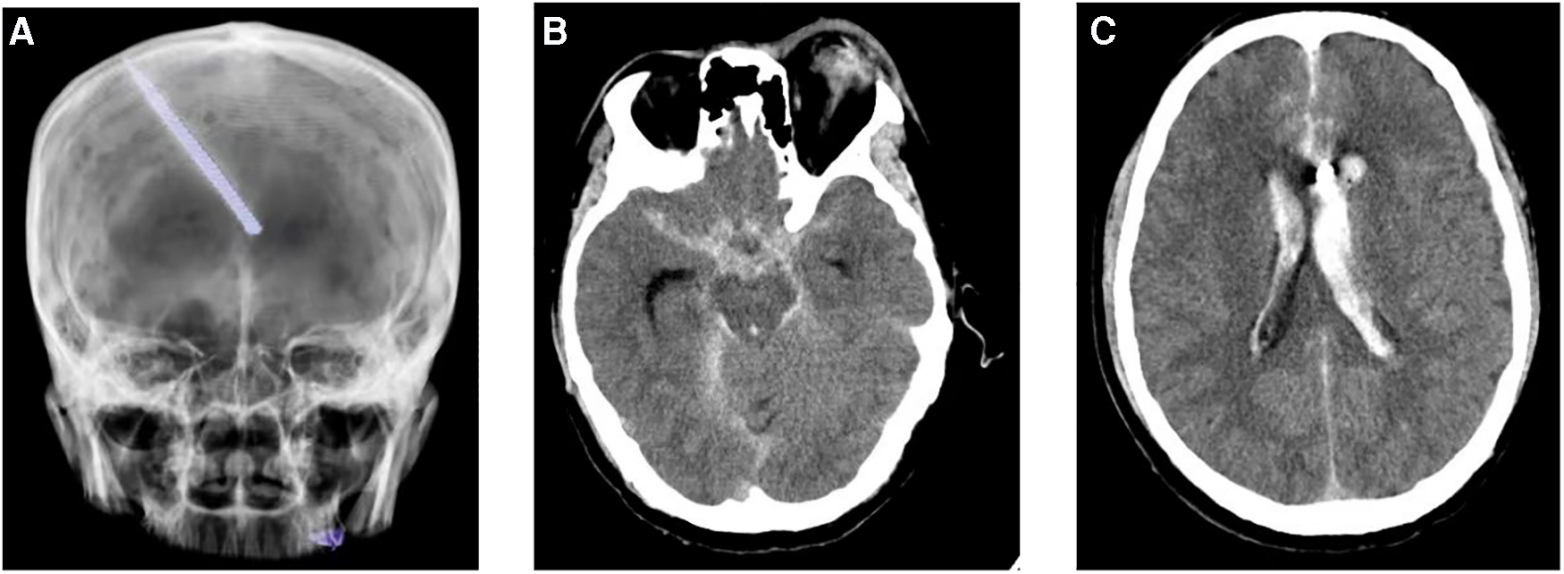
Computed tomography reveals a foreign body (metal strip) spanning the genu of the corpus callosum to the right parietal lobe (A); rupture of the left eyeball, subcutaneous hematoma of the eyelid, fracture of the top wall of the left orbit, and a small accumulation of gas in the left orbit (B); and contusion of the left frontal lobe with extensive subarachnoid hemorrhage (C).
